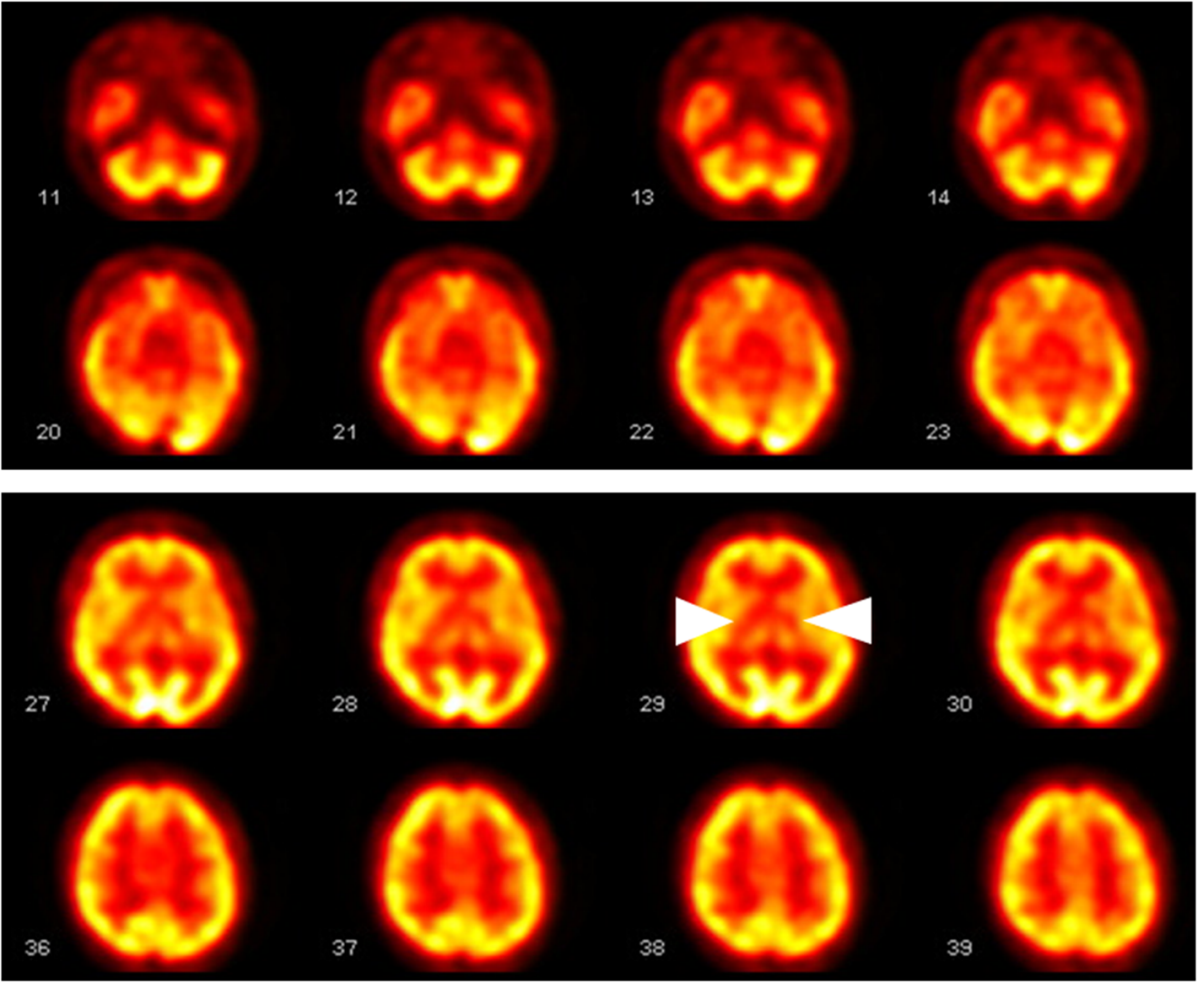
The features of cerebral perfusion SPECT. The presence of hypoperfusion in the bilateral basal ganglia and thalamic regions (*arrowheads*) as well as heterogeneous radiouptake in the bilateral cerebral hemispheres and cerebellar hemispheres corresponded to those calcified areas in brain CT
